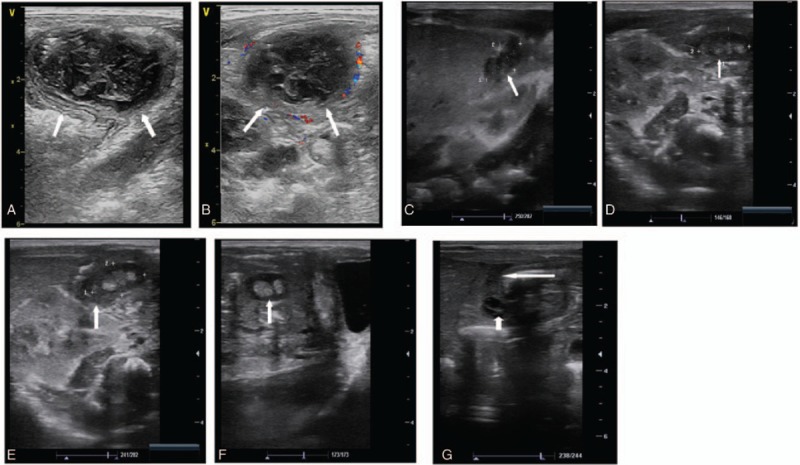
(A, B) A 17-day-old full-term female infant with abdominal distension, fever, and convulsions. Abdominal ultrasound shows a heterogeneous hypoechoic mass in the right lower quadrant (long- axis plane), 35 × 30 × 25 mm; and color doppler flow imaging shows a blood flow signal around the mass. (C, D) Preterm male infant with abdominal distension and tenderness in the right middle abdomen. Abdominal ultrasound shows a heterogeneous hypoechoic area in front of the right kidney and hyperechoic echoes within it. (E, F) Preterm male infant with gastric residuals and abdominal distension. Abdominal ultrasound shows a 14 × 7 mm heterogeneous echoic mass in the ileocecal area in the right lower quadrant with hyperechoic echoes inside. (G) A male infant in the neonatal intensive care unit with abdominal distension underwent abdominal ultrasound, which showed a stiff bowel in the right central abdomen, thickening of the bowel wall, mesenteric effusion, and low acoustic transmission (long arrow). A cystic echo was observed, which was considered an encapsulation (short arrow).
